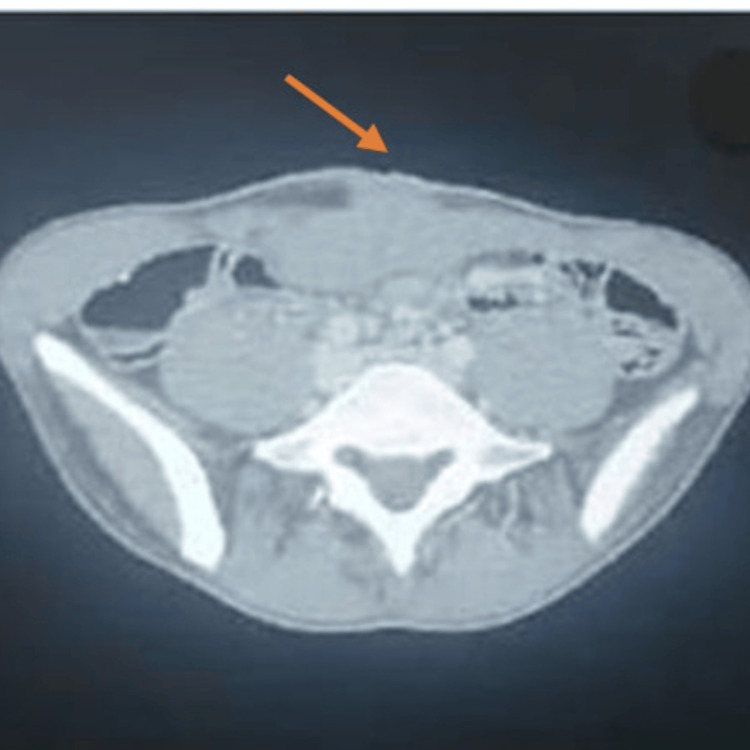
CT scan image demonstrating significant reduction in tumor size to 70 × 33 × 68 mm, as indicated by the arrow.
